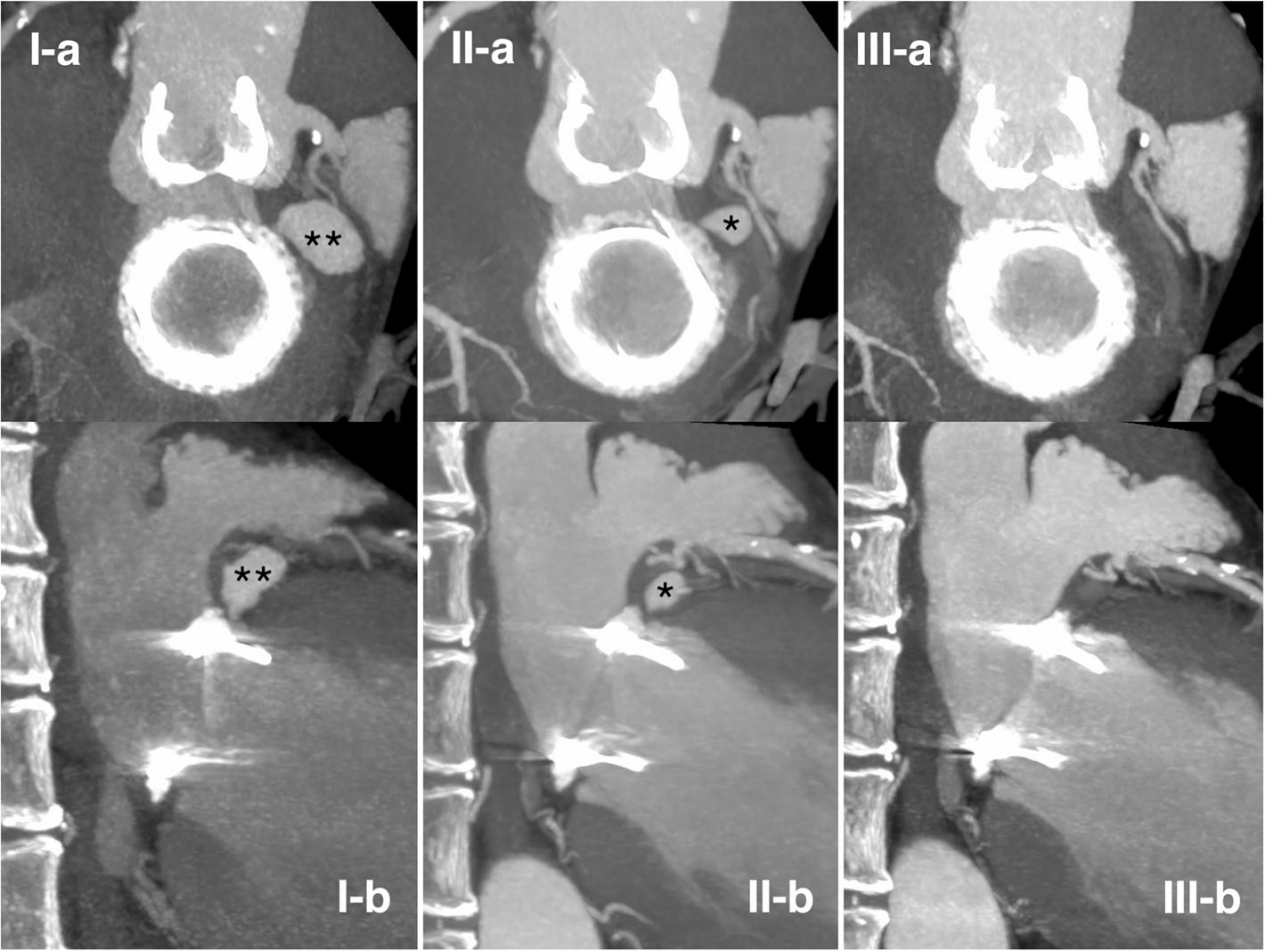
Pseudoaneurysm formation following atrioventricular groove hematoma. Changes over time are visualized by multiplanar reconstruction (MPR) of cardiac CT images. The upper row (I-a, II-a, and III-a) shows short-axis views and the lower row (I-b, II-b, and III-b) shows long-axis views. I-a and I-b: postoperative day 18, pseudoaneurysm and fistulous tract formation () arising from the posterior wall of the left ventricle just below the implanted mitral valve. II-a and II-b: three months postoperatively, regression in size of the pseudoaneurysm (*). III-a and III-b: six months postoperatively, complete resolution of the pseudoaneurysm
